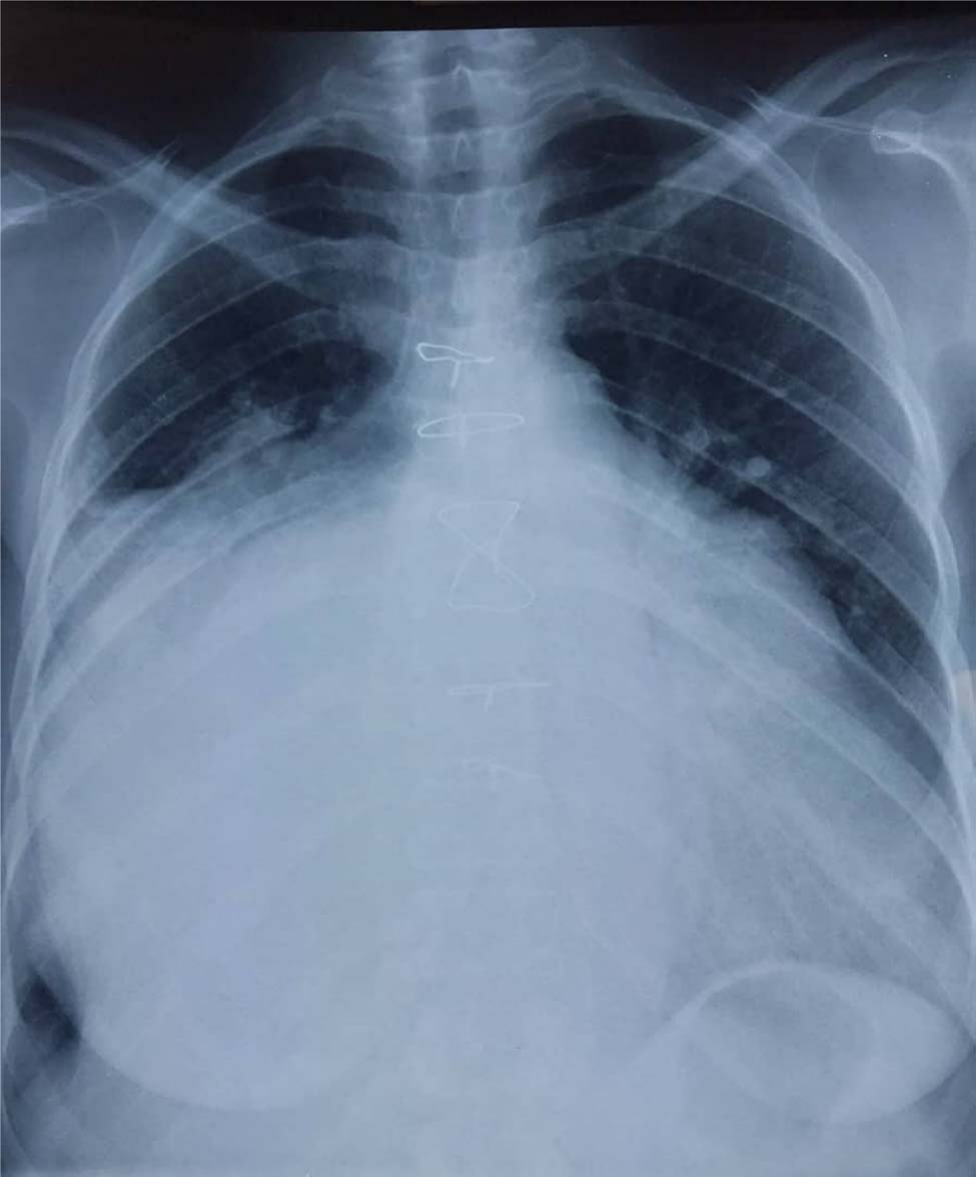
X-ray, increase the cardiothoracic index (cardiomegaly), subdiaphragmatic apex, medium convex left arch, and pleural effusion on the right side.
